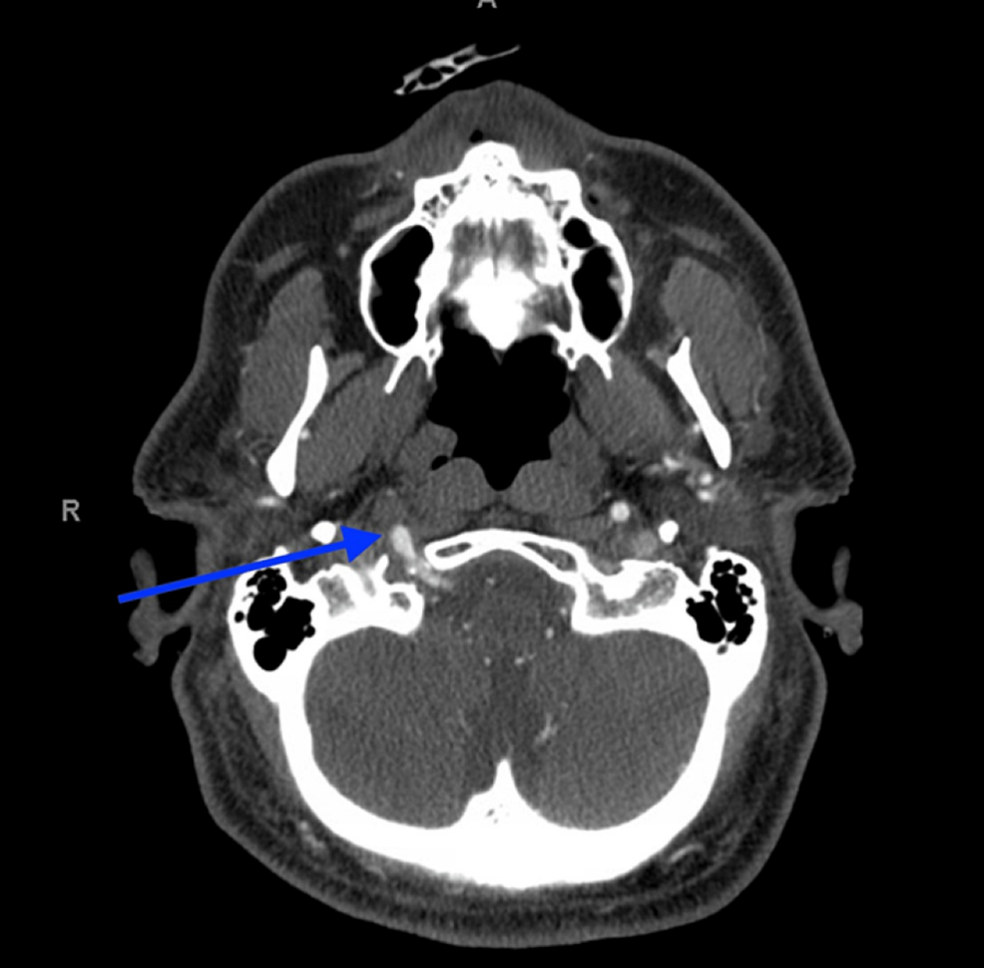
Computed Tomography Angiography Depicting the Right PPHA in Axial View
